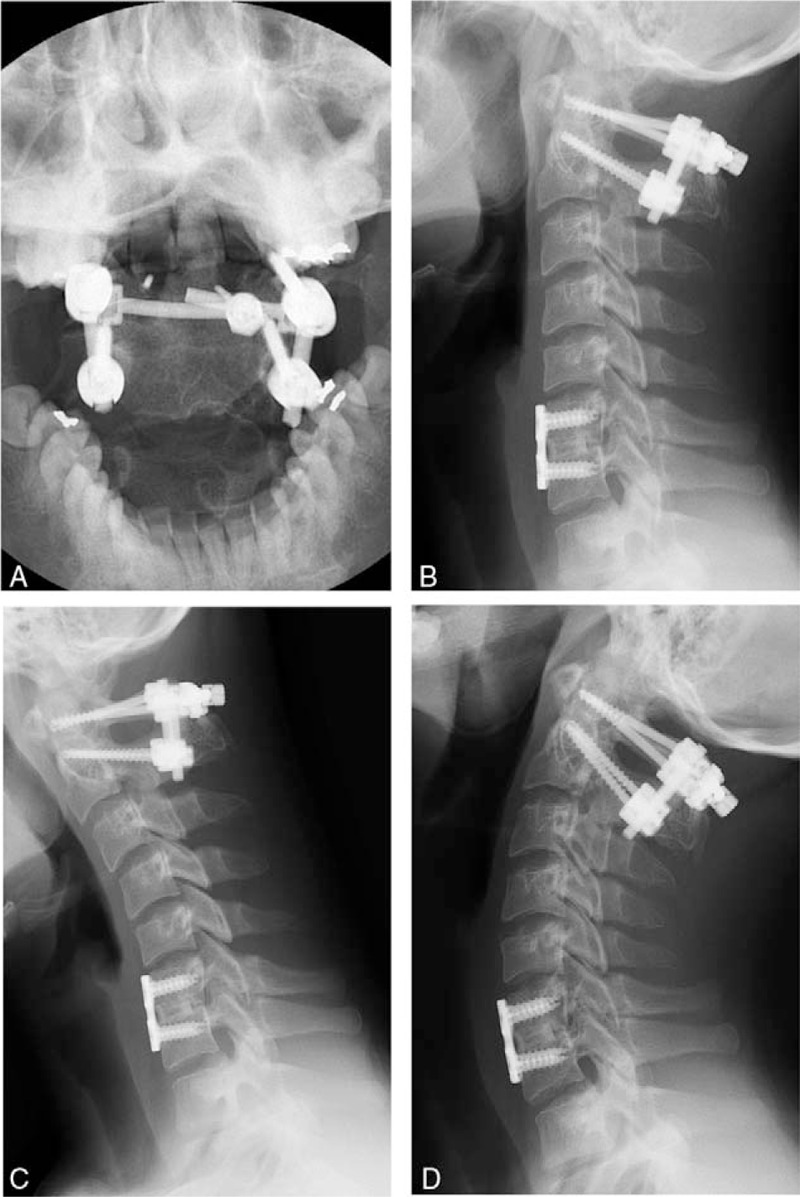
One-year postoperative plain radiographs show solid union of C1–2 and C6–7 without abnormal motion.
